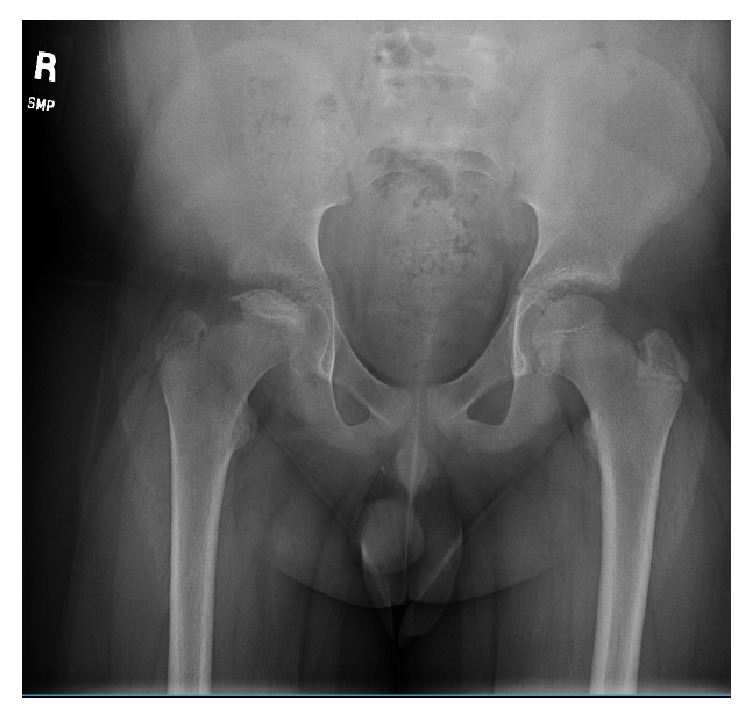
AP pelvis X-ray obtained 6 weeks from initial presentation which shows early femoral head collapse.
